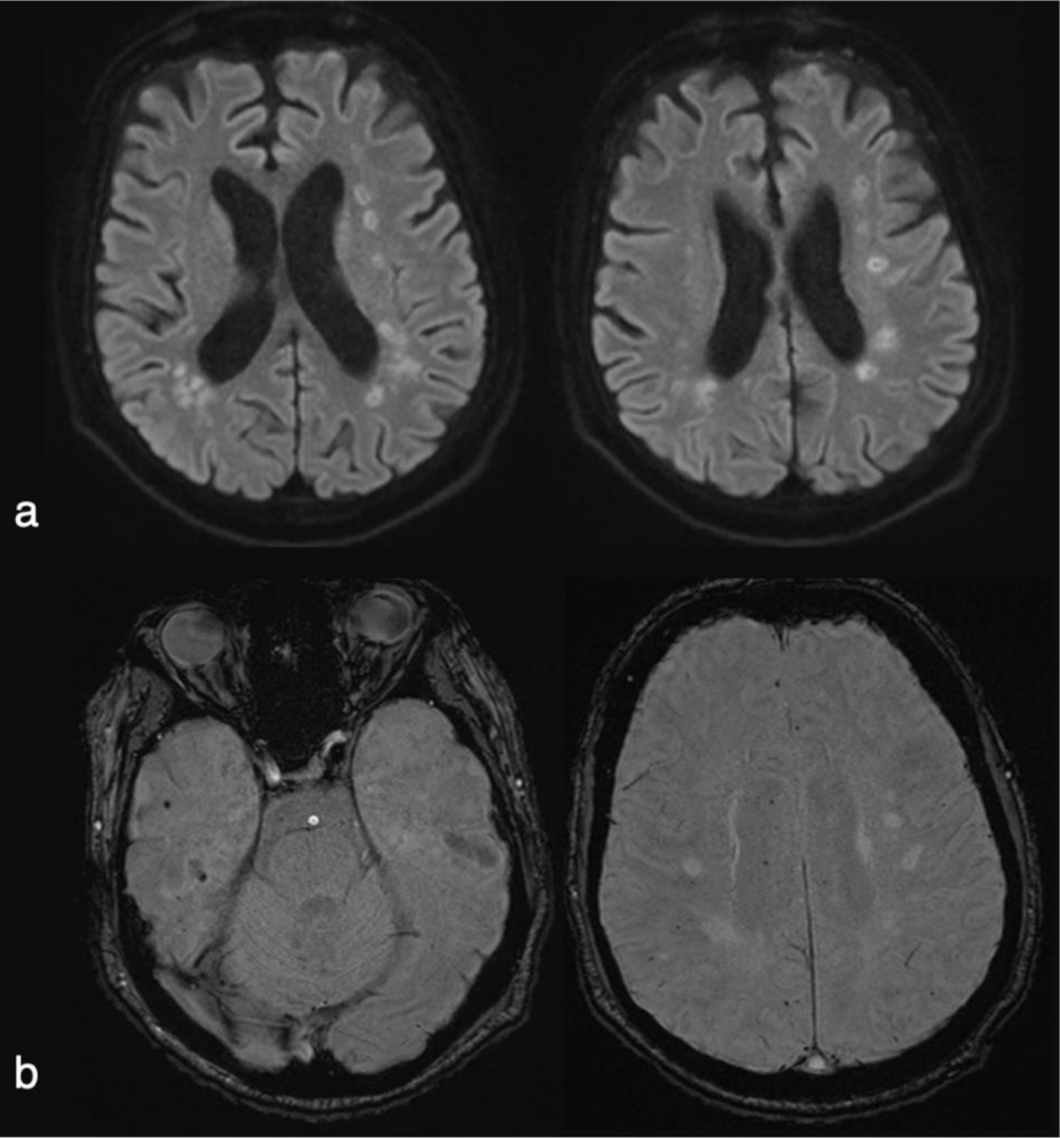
Brain MRI (a) DWI sequence: Multiple focal images predominantly periventricular and in both bilateral semioval centers, some of which present with restricted diffusion (not seen but they are hyperintense on T2/FLAIR and hypointense on T1) (b) SWI sequence: Hypointense punctiform foci that suggest microhemorrhages diffusely distributed in both supratentorial and infratentorial regions (cerebellar hemispheres and pons), in the corpus callosum and basal ganglia
